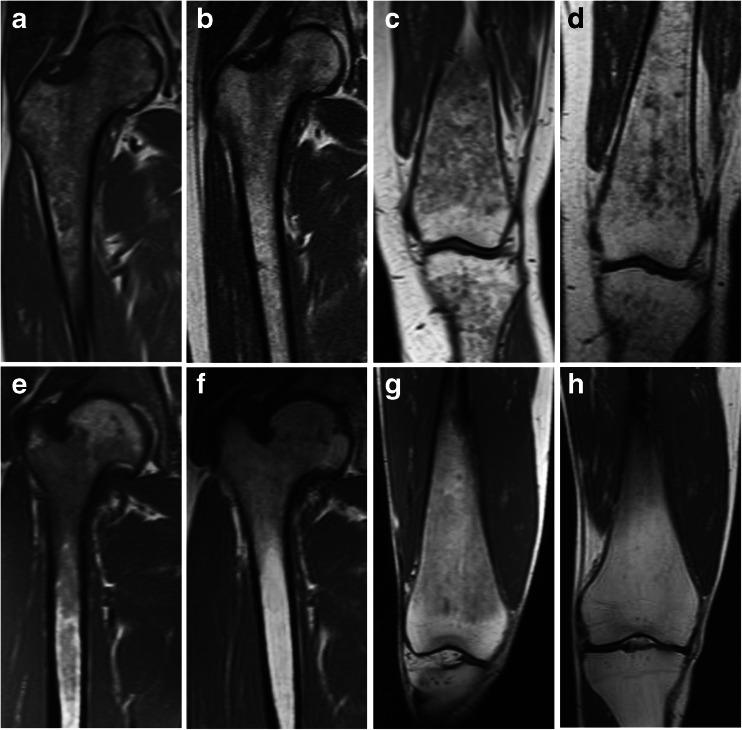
Dark marrow improvement in two patients after 4 years of eliglustat therapy. *Top* Coronal T1-weighted MR images of the proximal right femur of a 55-year-old female patient at baseline (a) and at 48 months (b) demonstrate decreased but persistent dark marrow in the femoral head epiphysis, greater trochanteric apophysis, and intertrochanteric region of the proximal femur. Dark marrow has also largely disappeared from the proximal femoral diaphysis. Coronal T1-weighted MR images of the distal left femur at baseline (c) and at 36 months (d) demonstrate substantially decreased dark marrow in the distal femoral diaphysis and metaphysis. *Bottom* Coronal T1-weighted MR images of the proximal right femur of a 30-year-old male patient at baseline (e) and at 48 months (f) demonstrate decreased dark marrow in the intertrochanteric region and proximal diaphysis of the femur. Coronal T1-weighted MR images of the distal right femur at baseline (g) and at 48 months (h) in the same patient demonstrate complete resolution of dark marrow in the distal femoral diaphysis and metaphysis with residual normal fatty marrow
